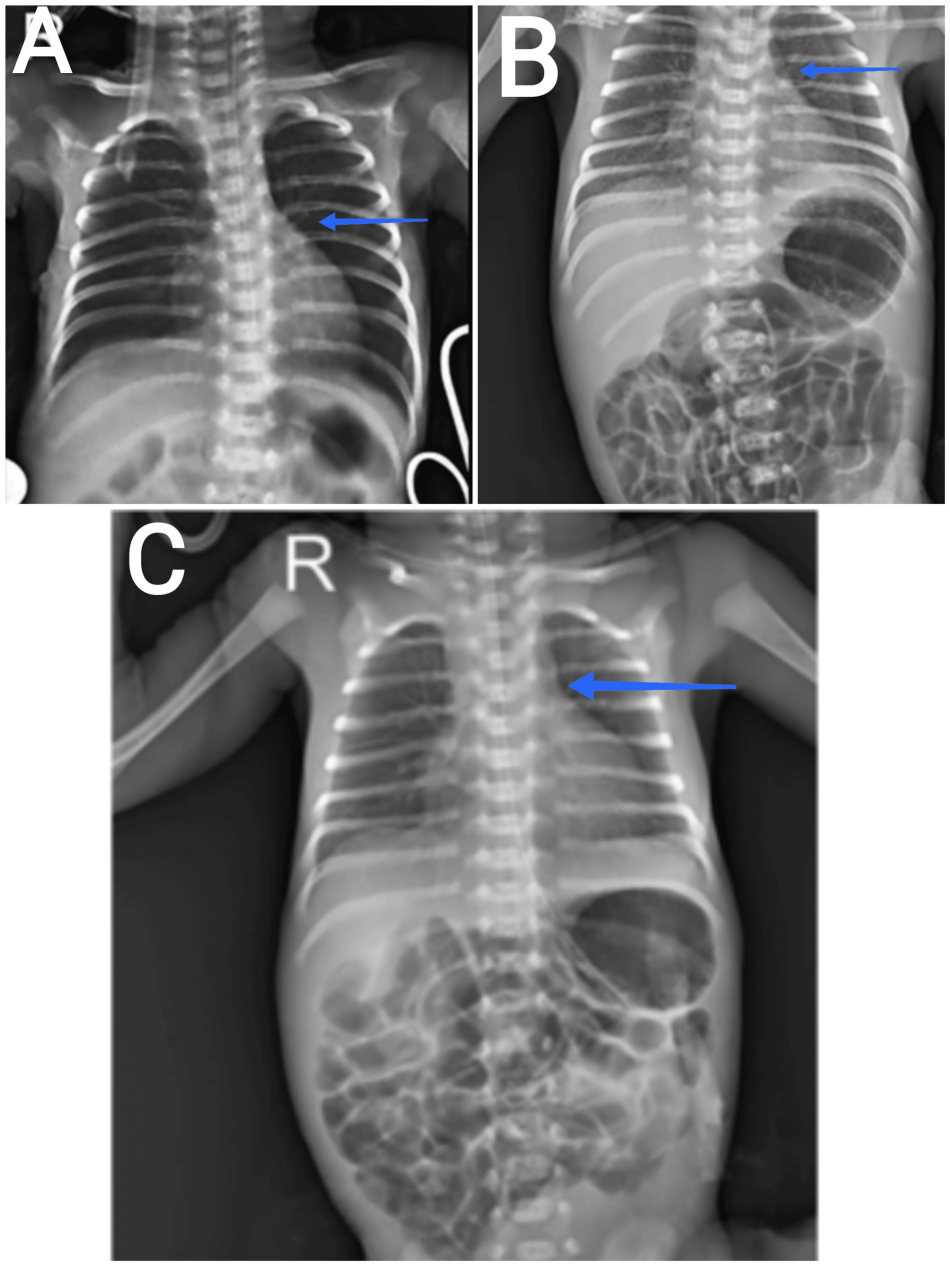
Chest radiograph of Baby A, Baby B, and Baby C The blue arrow in Figure 1A shows the decreased bronchovascular markings; Figure 1B and Figure 1C show increased bronchovascular markings
